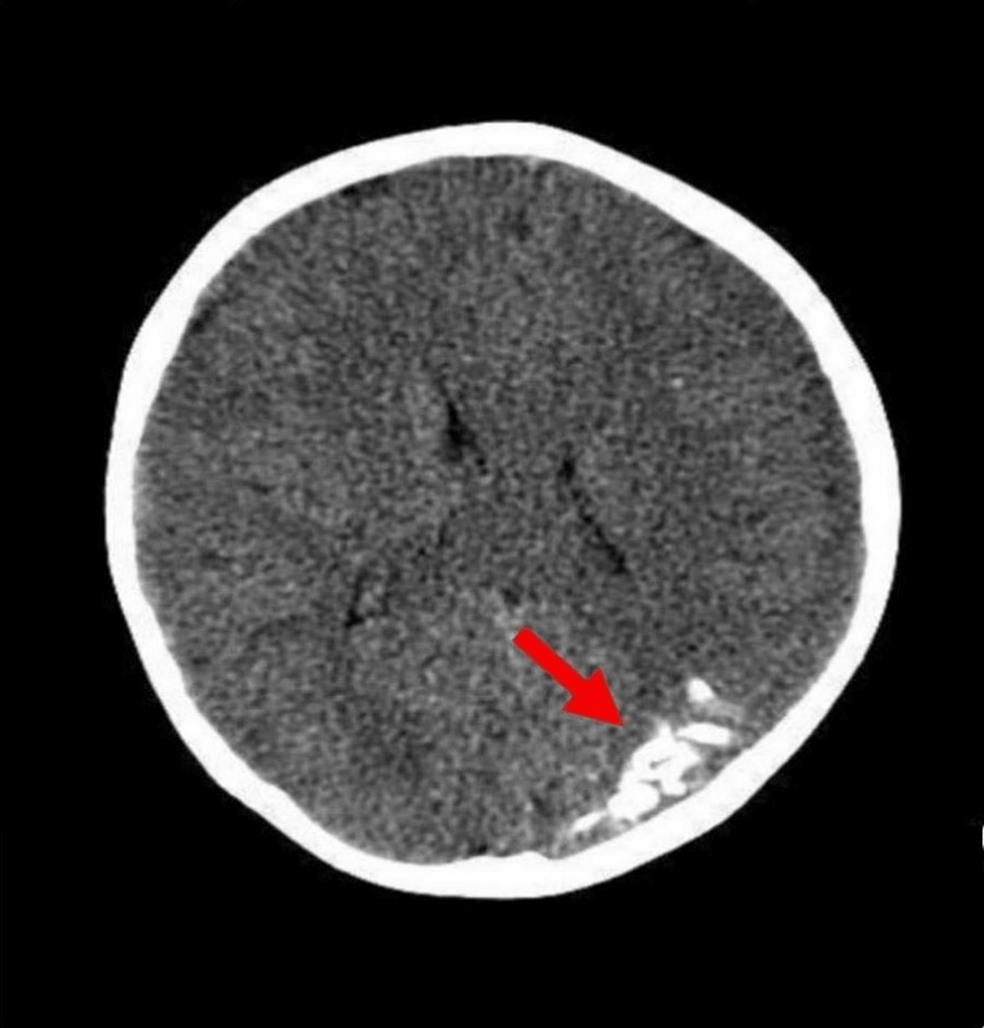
CT scan revealing gyriform calcifications in the left parieto-occipital region. The red arrow depicting the typical intracranial calcifications (tram track appearance).
